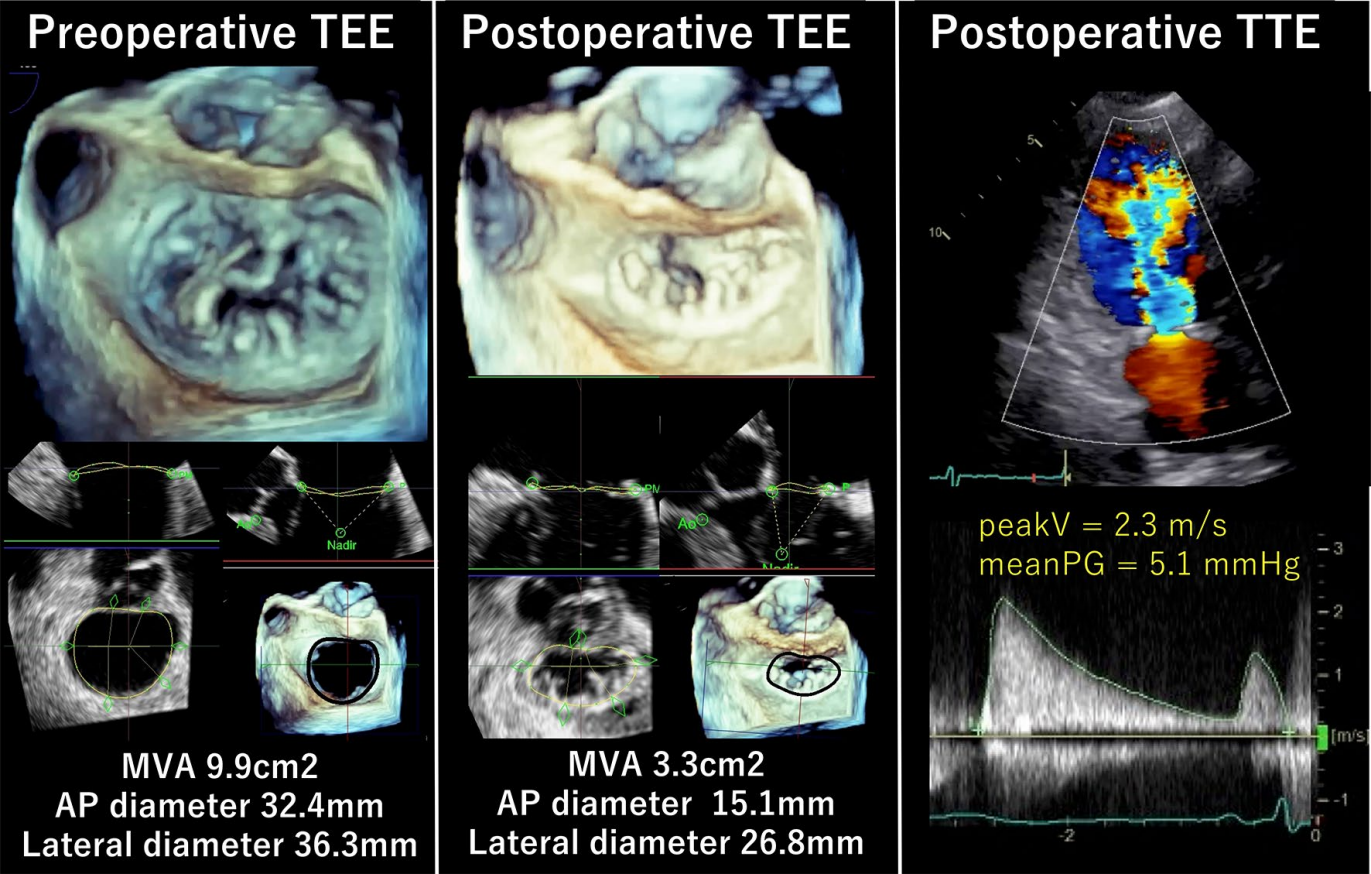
A representative case of postoperative functional mitral stenosis. A case of a 68-year-old female with P1 prolapse. The mitral valve annulus (MVA) was reduced from 9.9 cm^2^ to 3.3 cm^2^, and the area change ratio between pre- and post-MV repair was 67%. TEE; transesophageal echocardiography, TTE; transthoracic echocardiography, AP diameter; anterior to posterior diameter
